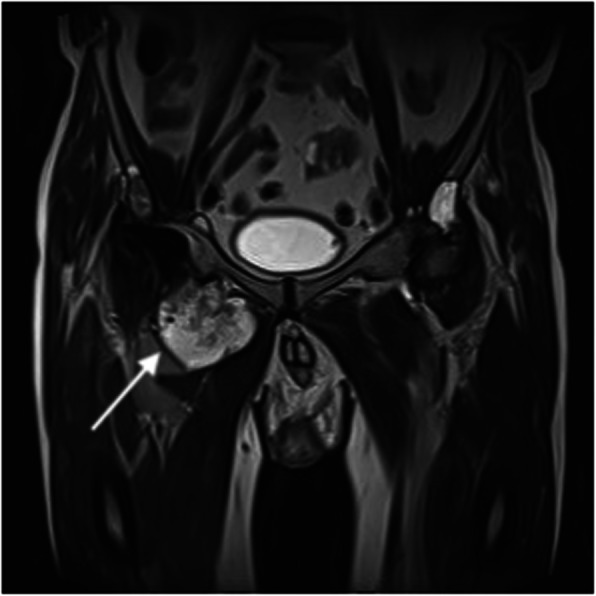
T1 MRI sequence of the pelvis showing the right total hip replacement with a large lobulated associated soft tissue mass arising in relation to the hip joint and the prosthesis (arrow), consistent with prosthesis-related pseudotumour
